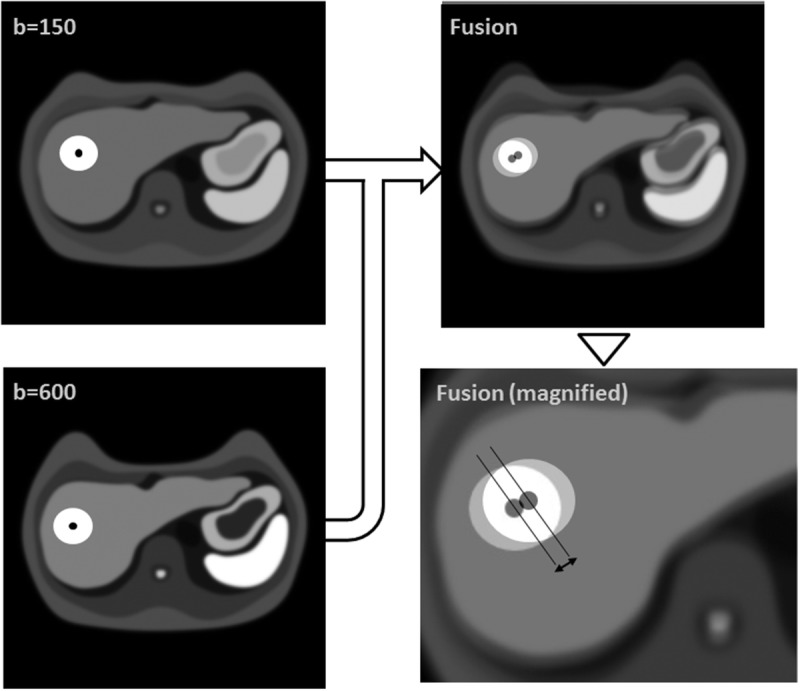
Calculation of the distance between the tumor gravity center on *b*-value of 150 and 600 s/mm^2^ DWIs. White circle indicate the tumor and black dot is located on the tumor gravity center. DWI with *b*-value of 150 s/mm^2^ was fused onto DWI with *b*-value of 600 s/mm^2^ (fusion image). Fusion image indicated the tumor location on *b*-value of 150 s/mm^2^ DWI was shifted from that on *b*-value of 600 s/mm^2^ DWI without registration. On the fusion image (magnified) the arrow indicates the distance between the tumor gravity center on *b*-value of 150 and 600 s/mm^2^ DWIs. The distance should be decreased if registration corrected mis-registration between the two DWIs.
